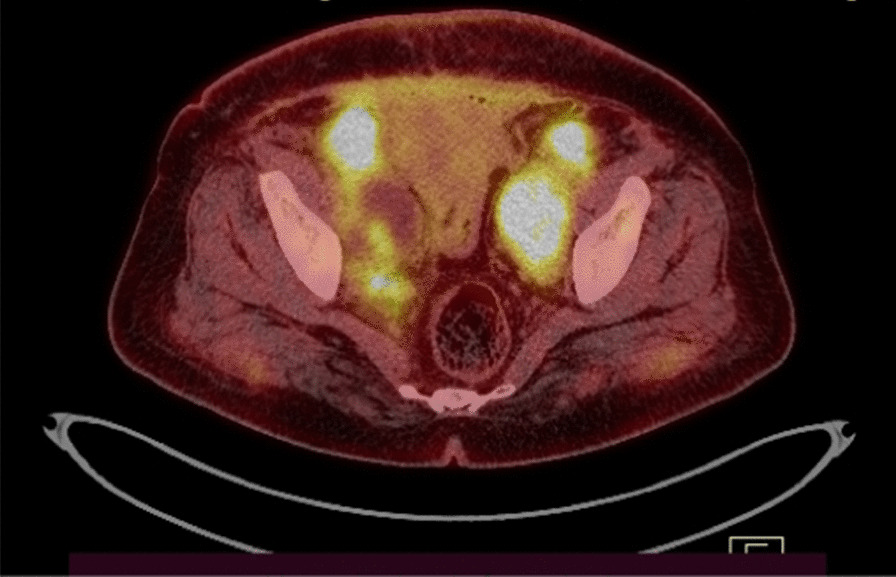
Positron emission tomography–computed tomography 5 months after the first-line chemotherapy. Lower abdominal wall and pelvic peritoneal tumoral involvement
